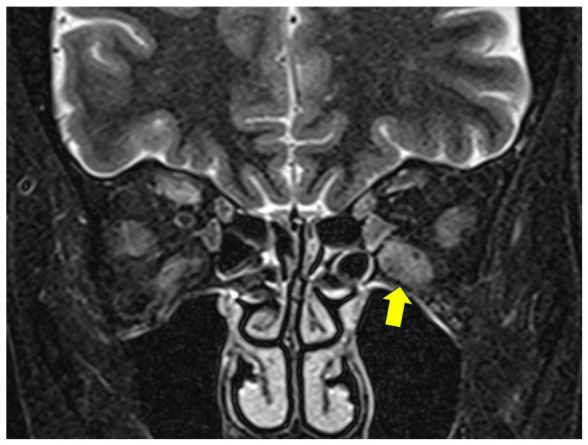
Inflammation of the left inferior rectus muscle shown as high signal intensity on a short tau inversion recovery (STIR) magnetic resonance image (arrow).
